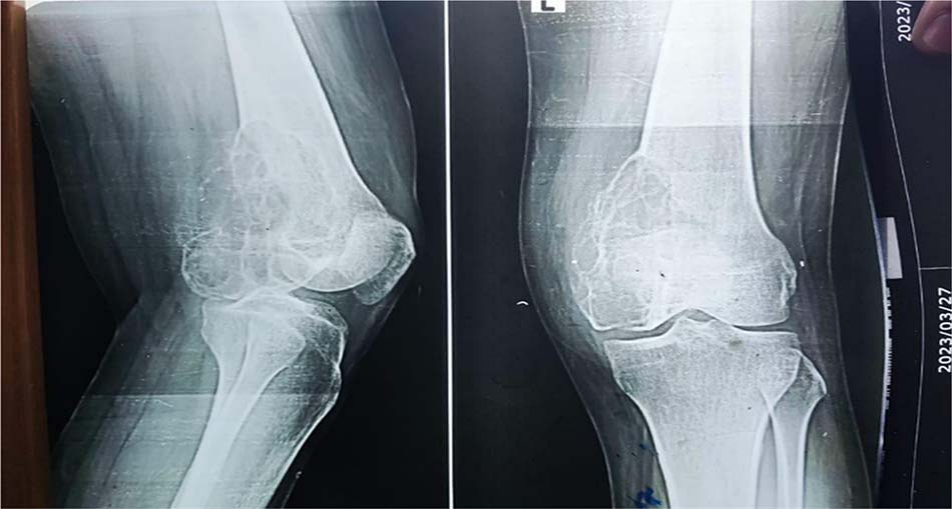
The X-ray shows a distal femur osteolytic lesion with a soap bubble appearance, a narrow zone of transition, a soft tissue component, and a sparing knee joint in the X-ray taken 6 months before pregnancy.
